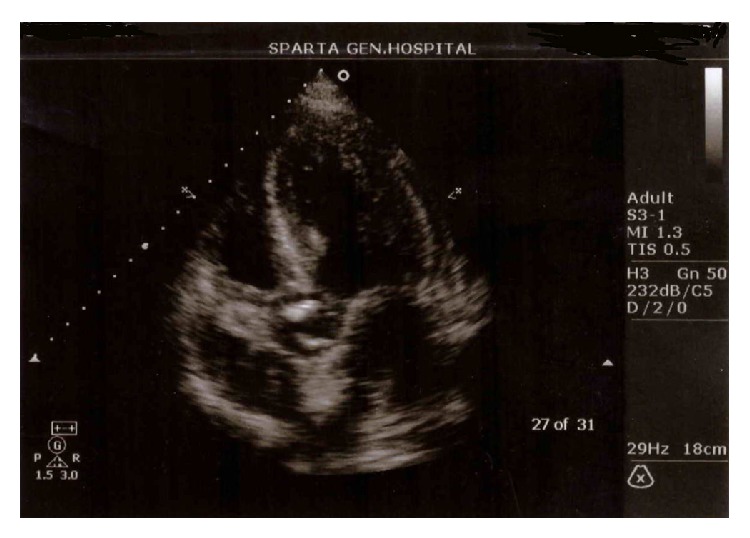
Transthoracic echocardiogram of the patient showing bicuspid aortic valve with vegetations.
